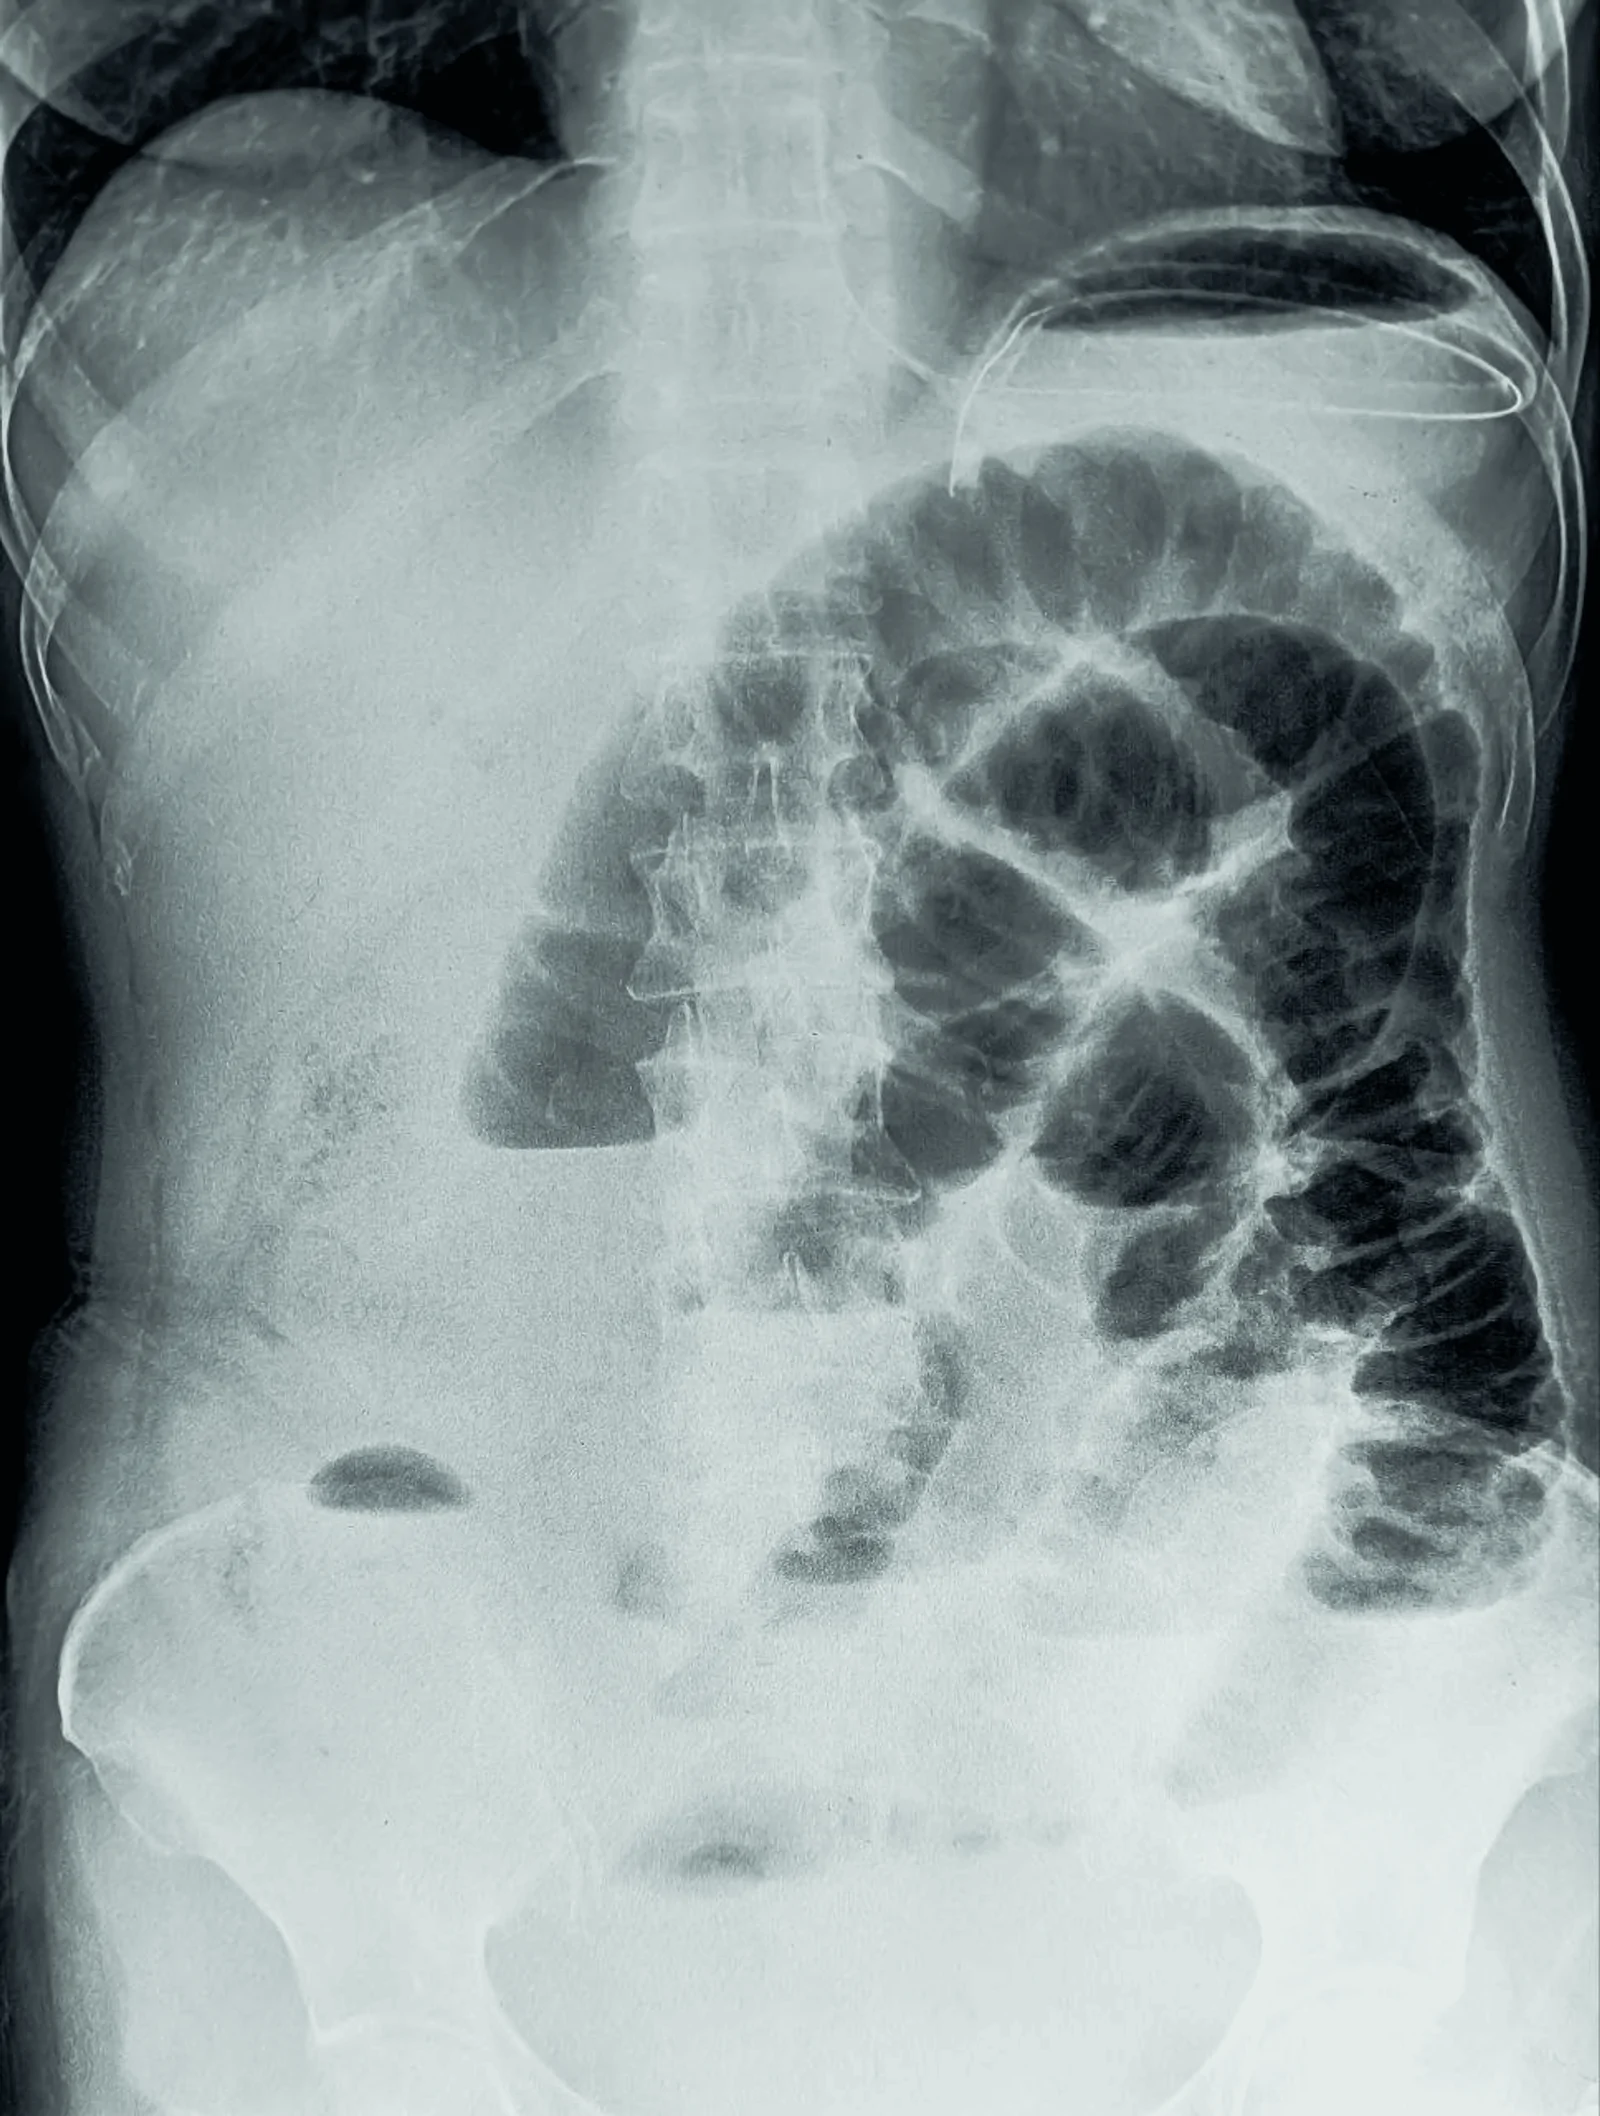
Digital straight X-ray of abdomen in erect posture consistent with the features of small-bowel obstruction
